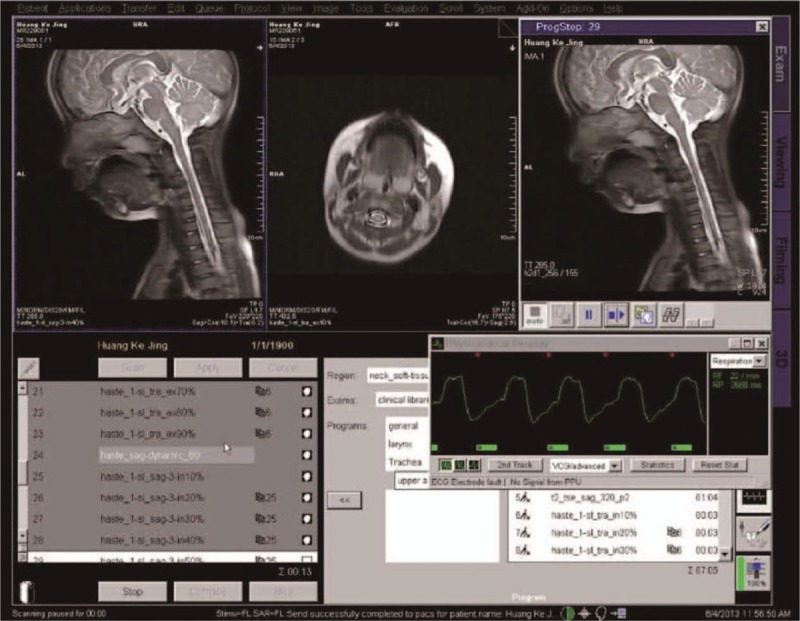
Panel for setting the parameters of the MRI-respiratory gating technology.
